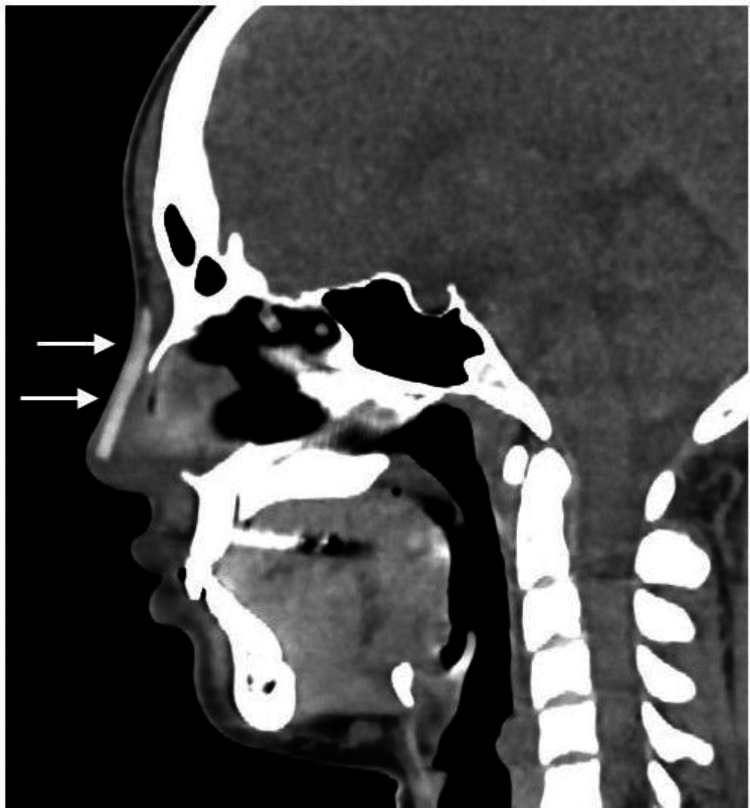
A computed tomography scan of the face A rhinoplasty artifact on the nasal dorsum (white arrows), without surrounding fluid accumulation, is revealed.
